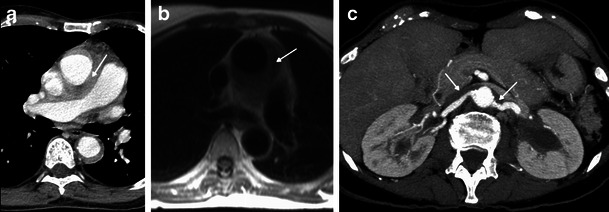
Axial CT-enhanced (a) and axial HASTE MR (b) images of the mediastinum and axial MIP CT (c) image of the upper abdomen in a 73-year-old male. There is periaortic and mediastinal soft tissue infiltration (arrow) and also infiltration of the origin of the renal arteries (arrows) and of the left renal sinus, with mild hydronephrosis (arrow)
